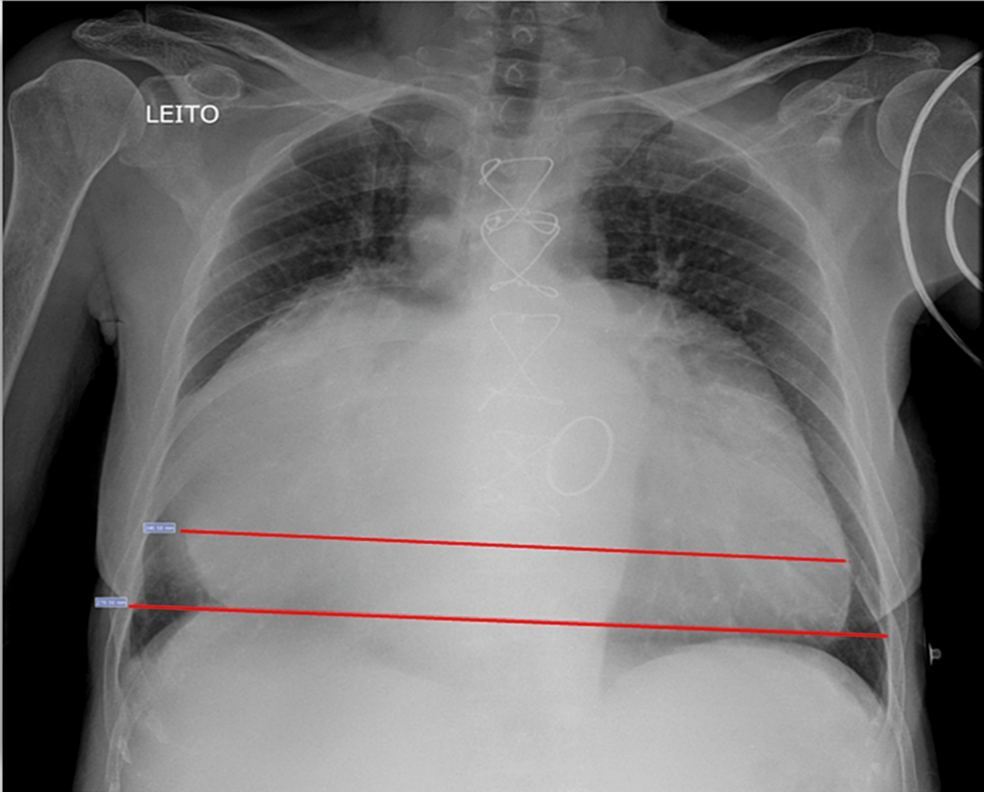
Chest X-ray taken upon admission showing severe cardiomegaly. Measured cardiothoracic ratio is 0.89.
